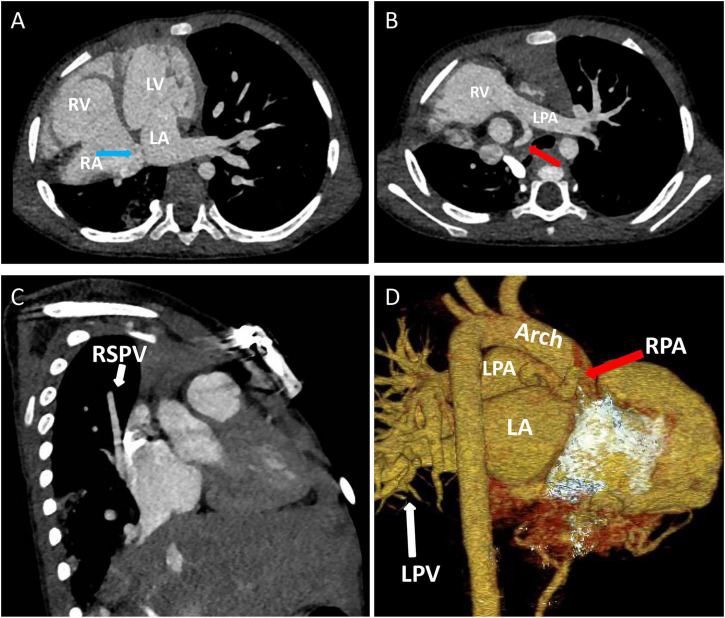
Contrast-enhanced CT of the chest and three-dimensional cardiac computed tomography angiography: (A) Atrial septal defect location, ∼9 mm in size (blue arrow). (B–D) The right pulmonary artery (red arrow) and right superior pulmonary vein are hypoplastic (white arrow). LA, left atrium; LPA, left pulmonary artery; LPV, left pulmonary vein; LV, left ventricle; RA, right atrium; RPA, right pulmonary artery; RSPV, right superior pulmonary vein; RV, right ventricle.
